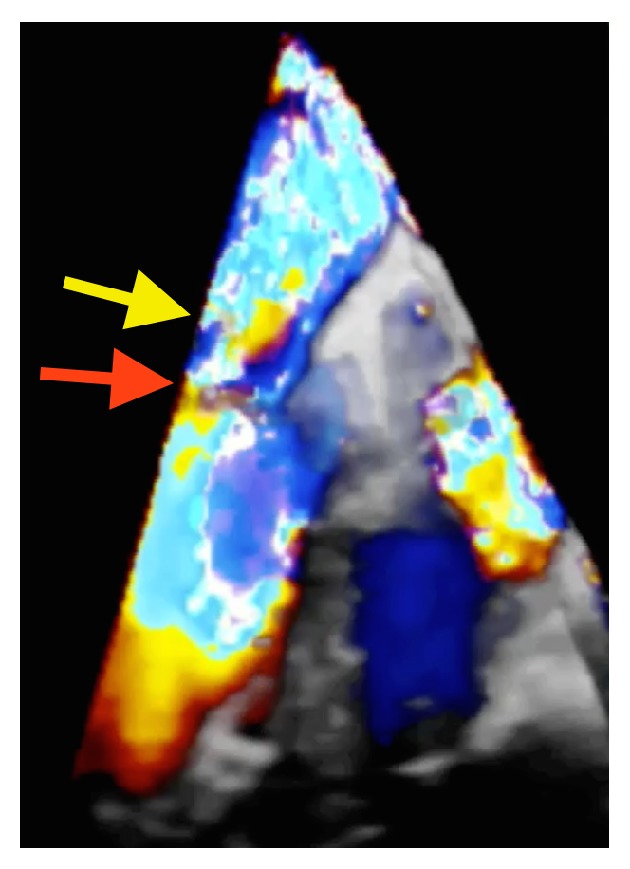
Color 3D TEE image on an Apical 4 Chamber view shows the impressive lateral jet (red arrow) originating from the perforation on the P2 scallop of posterior mitral leaflet. The less impressive central jet of mitral regurgitation is partially seen in this image (yellow arrow).
